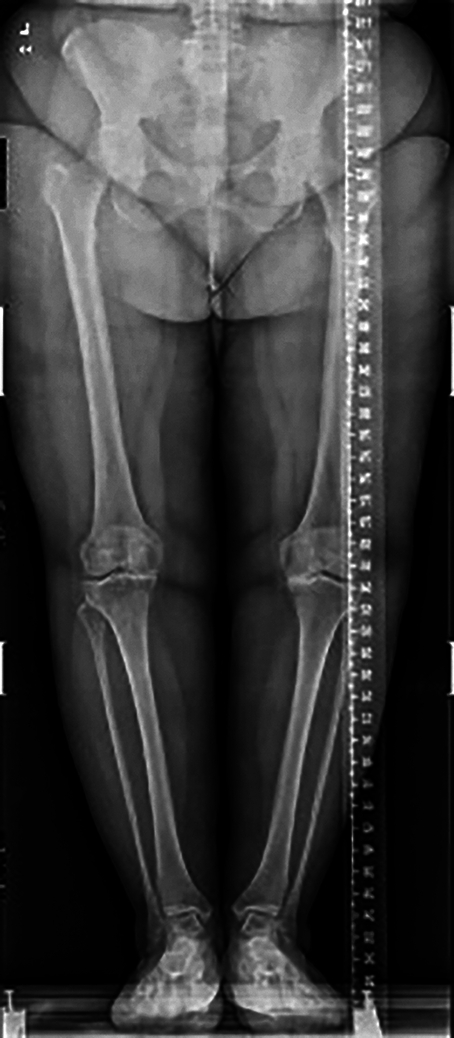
Plain films revealing varus deformities and osteoarthritis in both knees.
